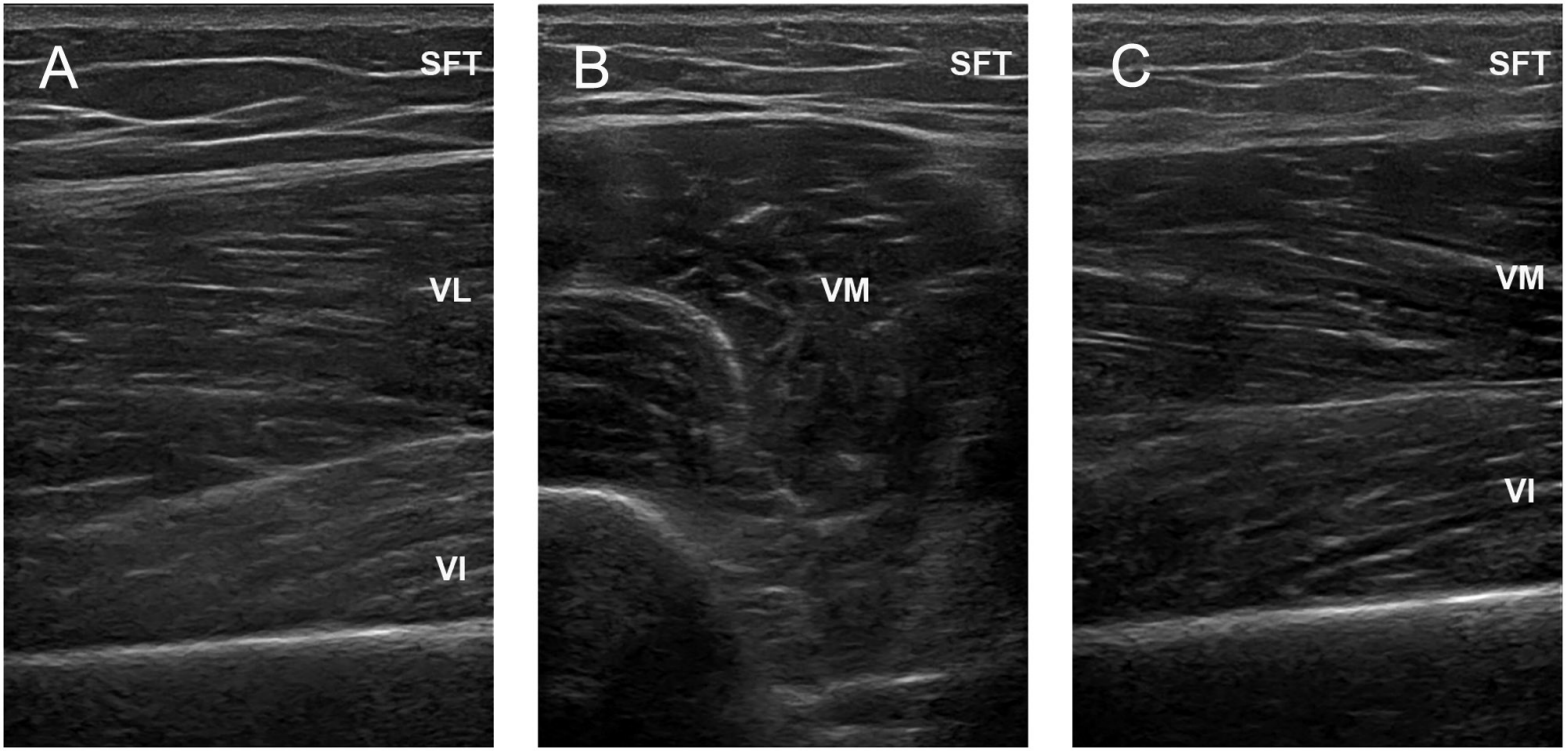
Examples of muscle ultrasound images in the non-CTM group. A. Longitudinal section of the VL. B. Cross section of the VM. C. Longitudinal section of the VM. CTM, chronic thyrotoxic myopathy; SFT, subcutaneous fat thickness; VI, vastus intermedius; VL, vastus lateralis; VM, vastus medialis.
